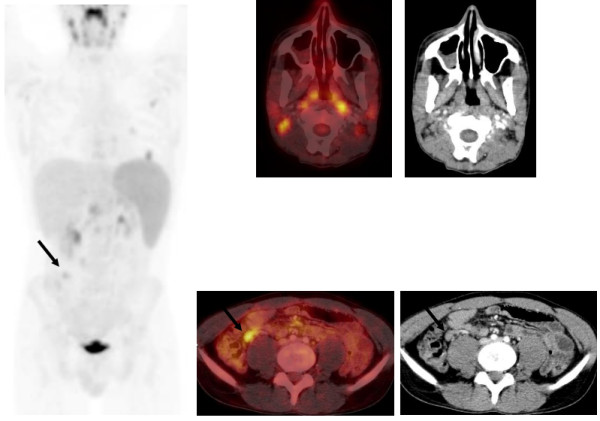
The second interim F^18^-fluorodeoxyglucose-positron emission tomography/computed tomography after cycles of cisplatinum, cytarabine, and dexamethasone, and etoposide, ifosfamide and methotrexate, showing decreased metabolic activity in the three lesions in the left lung indicating partial response. However, new pathological increased fluorodeoxyglucose uptake is seen in multiple bilateral cervical plus axillary lymph nodes and furthermore increased metabolic activity in the spleen and in a lymph node within the high-right para-aortal and in a lower-right mesenteric lymph node (black arrows). Virus serology was positive for Epstein–Barr virus.
